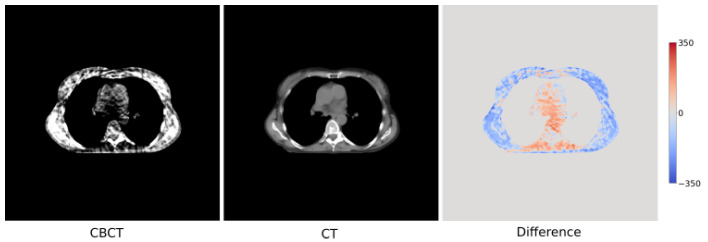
CBCT (left) and CT (middle) scans for a randomly chosen patient. Differences between the two scans with a HU range of [−300, 300] are also shown (right).
